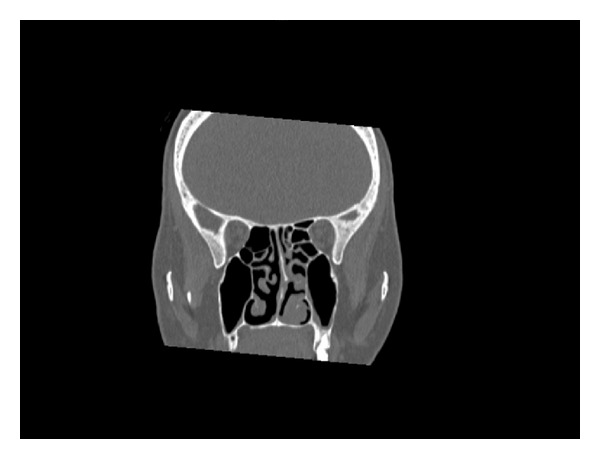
Coronal section of paranasal sinus CT scan of the patient after 1 month.
